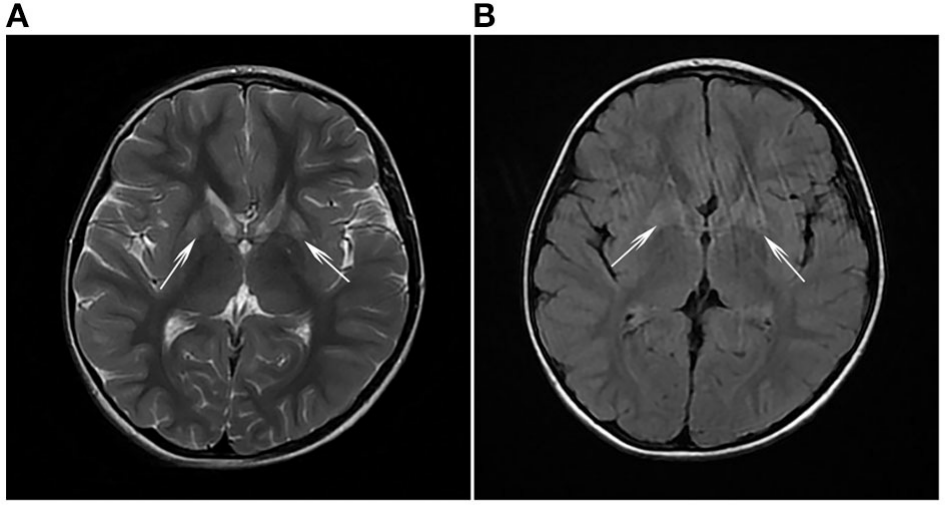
(A) T2-weighted imaging (T2WI) and (B) fluid-attenuated inversion recovery (FLAIR) analyses revealed symmetrical flake abnormal signal shadows in bilateral basal ganglia (white arrows).
